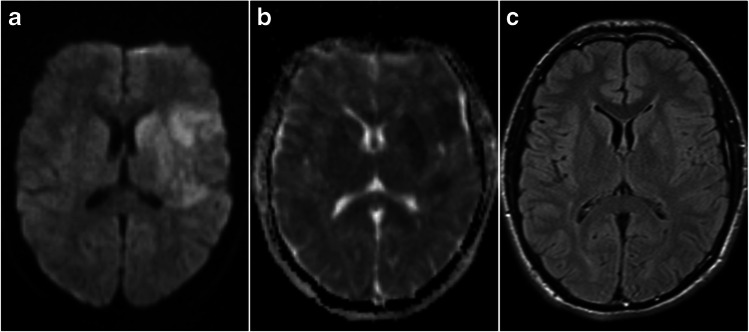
DWI and FLAIR images acquired in the phase of intracellular oedema. a The image shows an increased signal on DWI with b a corresponding signal drop on ADC and c a preserved normal signal on FLAIR
